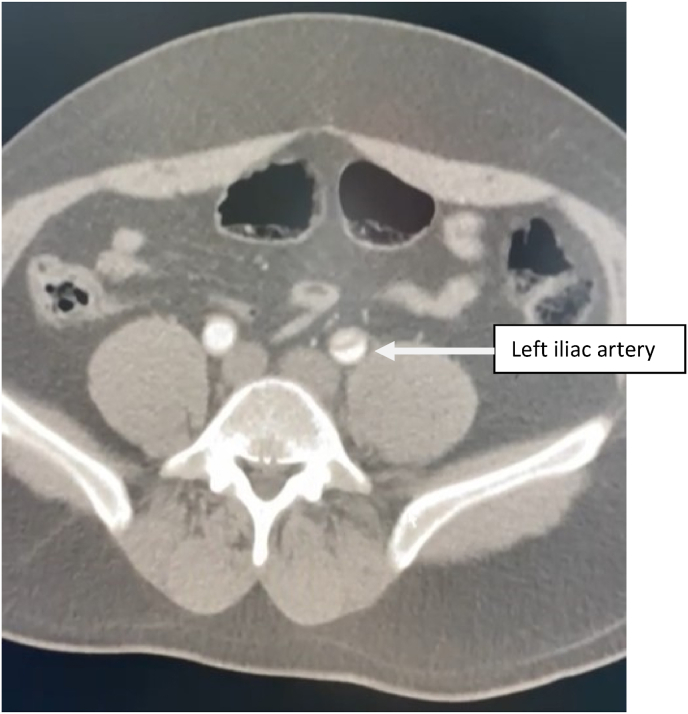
CT scan image showing the extension of the dissection to the iliac arteries.
